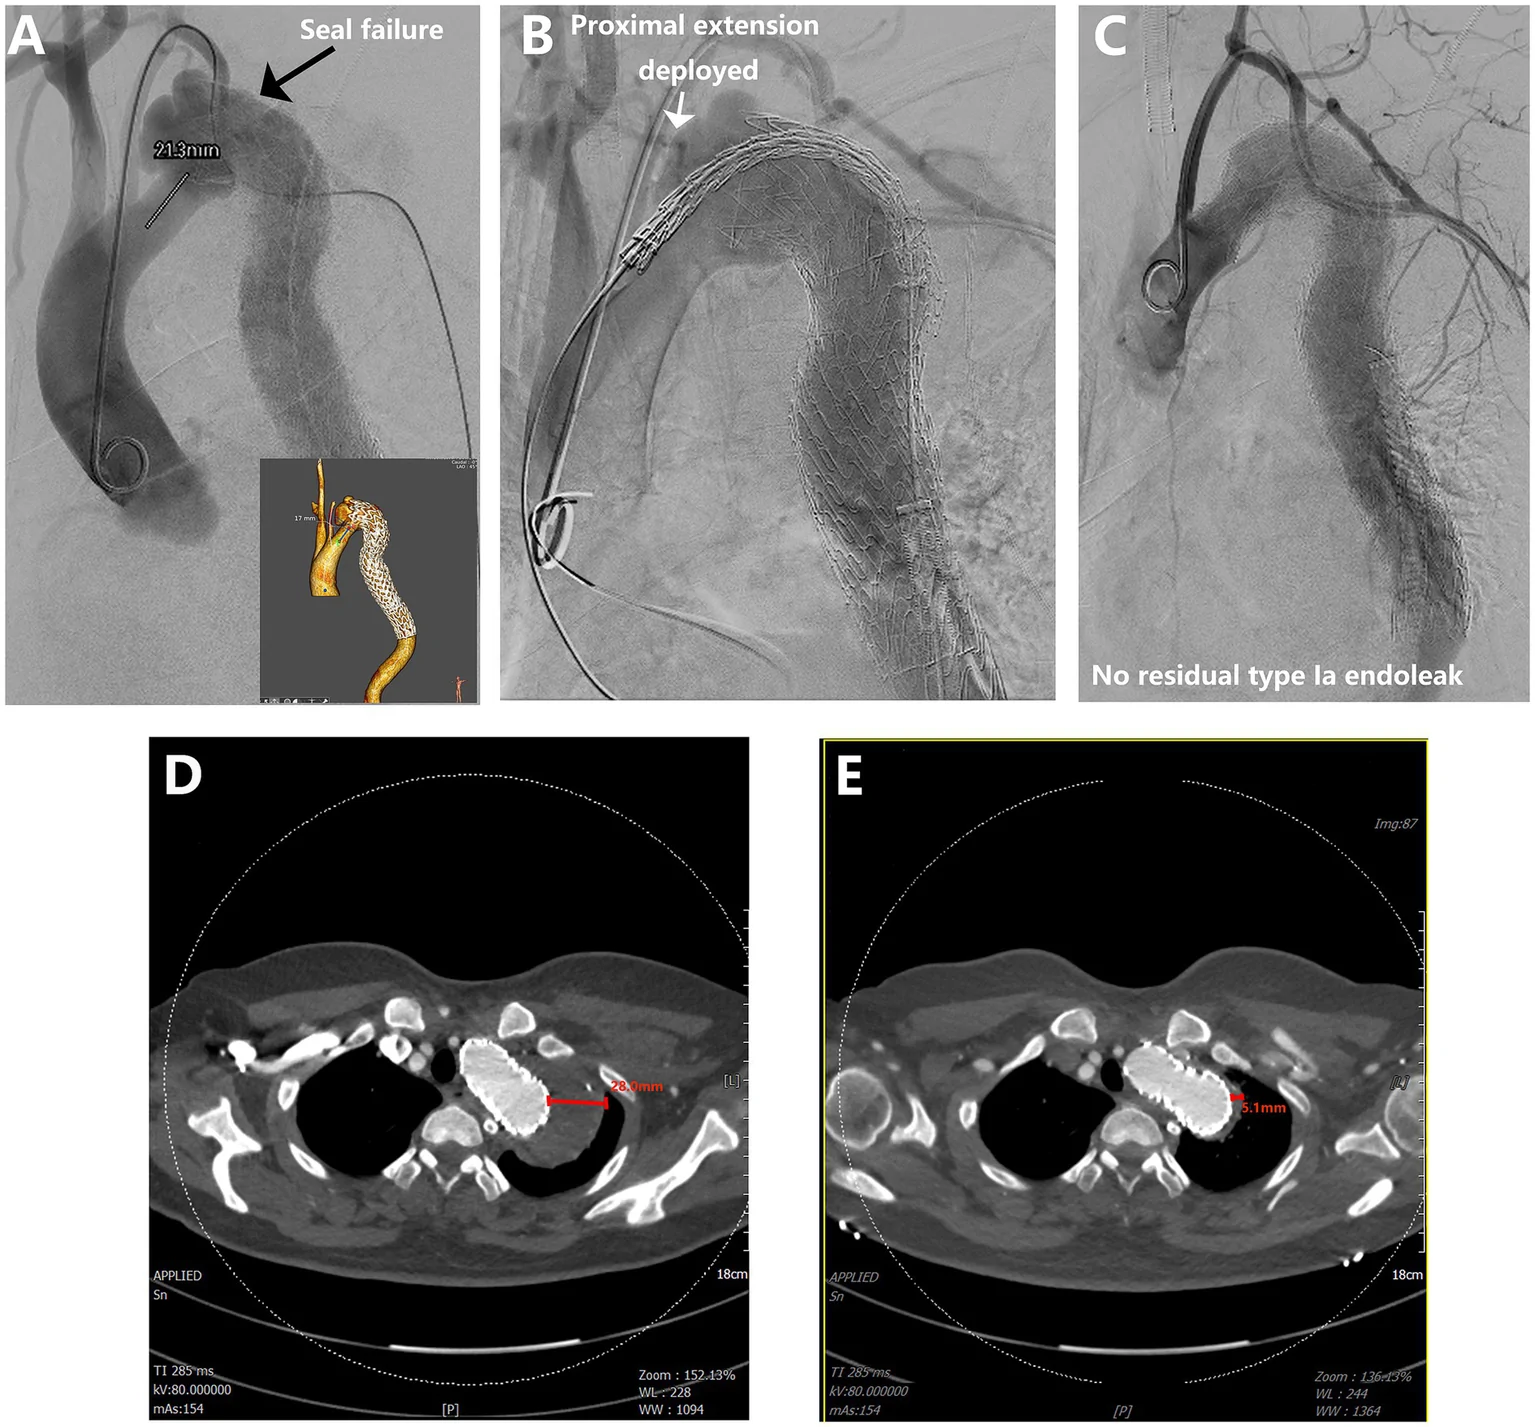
Early proximal seal failure with interval distal endograft displacement and endovascular bailout. (A) Postoperative day-9 angiography showing proximal seal failure and a 21.3-mm gap relative to the distal edge of the LCCA ostium. The inset reconstruction reports 17 mm of distal displacement, although identical-projection immediate postoperative imaging was unavailable. (B) Proximal extension deployed immediately distal to the LCCA. (C) Completion angiography showing no residual type Ia endoleak. (D,E) Axial follow-up images at 1 month and approximately 12 months showing reduction in radial excluded-sac thickness from 28.0 mm to 5.1 mm. These values are not maximum sac diameters. CTA, computed tomography angiography; LCCA, left common carotid artery.
